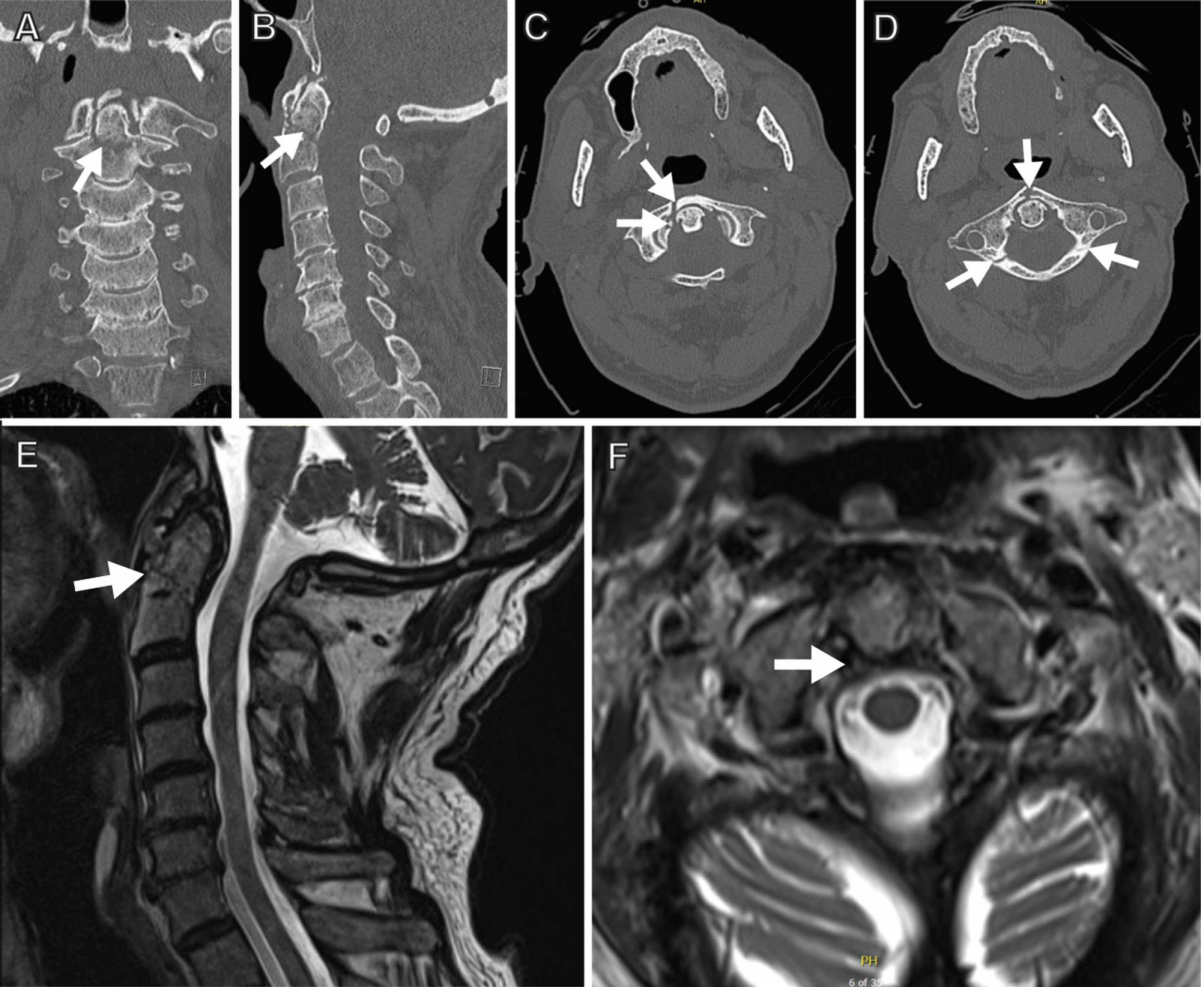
Preoperative images (A, B) coronal (A) and sagittal (B) CT views of the cervical spine demonstrate a type II odontoid fracture. (C, D) axial views demonstrate a chronic-appearing Jefferson fracture (chronic Jefferson fracture indicated by arrows). (E, F) T2-weighted MRI sagittal (E) and axial (F) images demonstrate the odontoid fracture (arrow in E) and an intact transverse ligament (arrow in F) CT: computed tomography; MRI: magnetic resonance imaging
